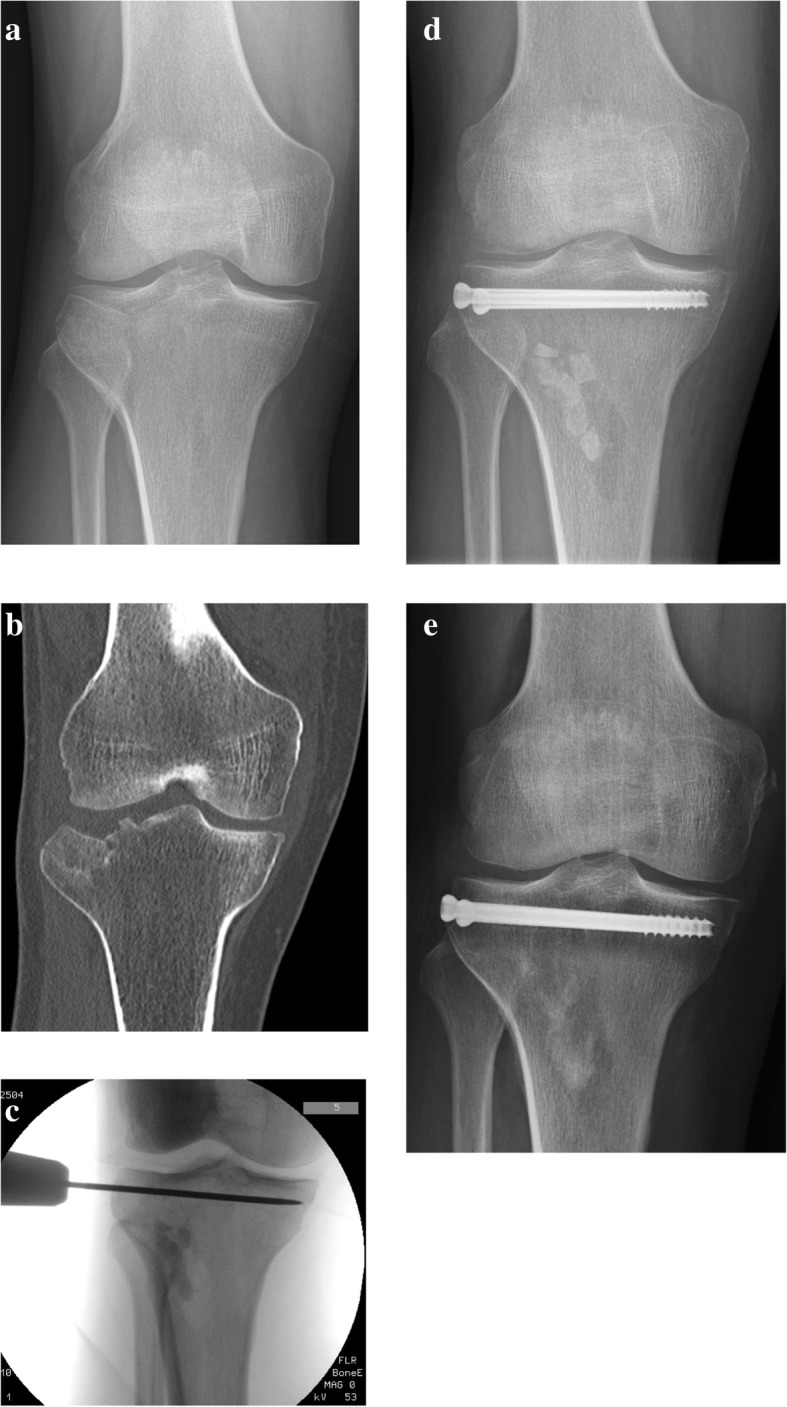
a Pre-operative anteroposterior x-rays of a patient with a split compression tibial plateau fracture (Schatzker type III). b Coronal section of computerized tomography scan. c Intra-operative fluoroscopy showing temporary fixation with a K-wire and metaphyseal bone defect filled with bone substitute grafting. d Post-operative anteroposterior x-rays of two cannulated screws fixation. e Postoperative 12 months’ anteroposterior x-rays showing good radiological results and initial resorption of the bone graft
